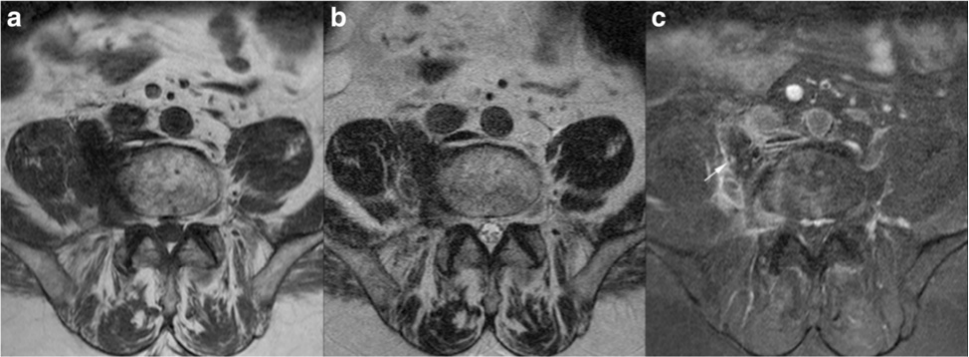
Axial MRI shows the lesions as hypointense on T2 (a) and T1 (b), with peripheral enhancement (arrow) on T1 fat sat after injection of contrast (c)
